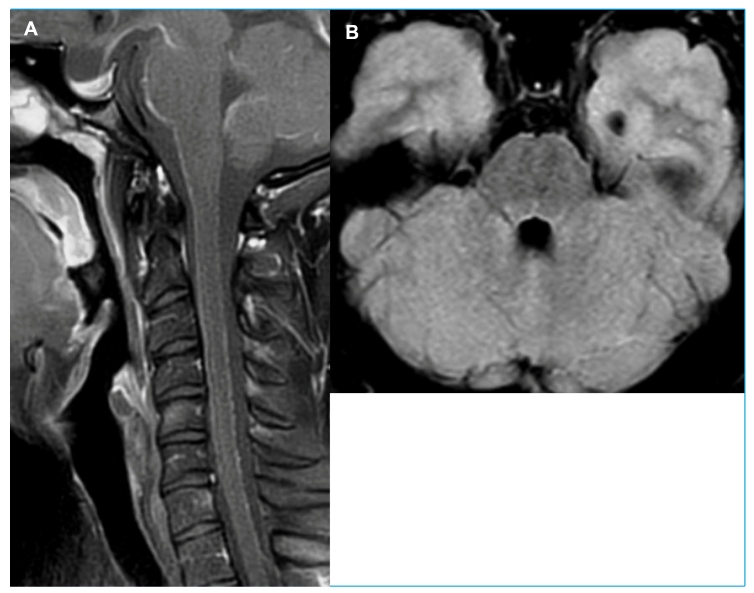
Sagittal T1-weighted fat suppressed shows diffuse leptomeningeal thickening along the surface of the spinal cord and brainstem. FIGURE 1B: Axial FLAIR image shows linear hyperintensity along the surface of the pons, indicating leptomeningeal thickening.
